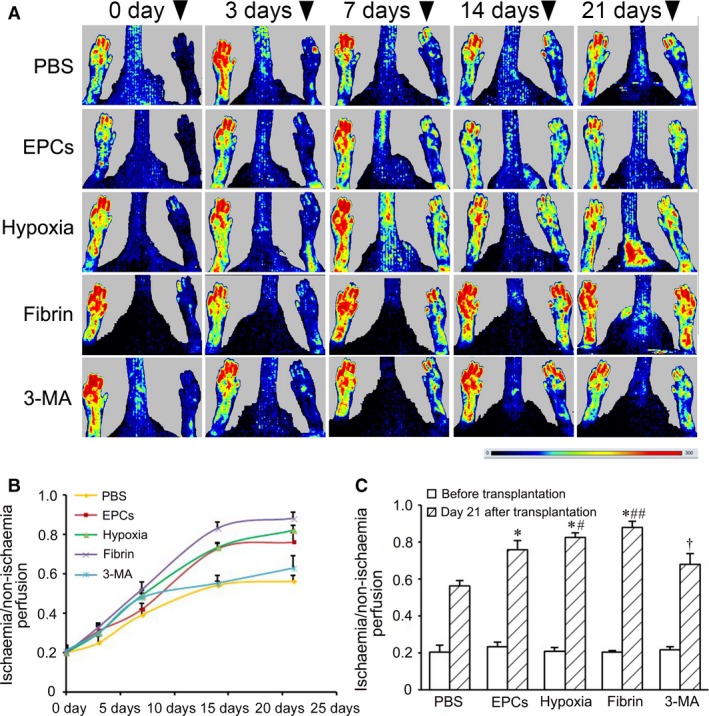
Improvement of blood perfusion in the ischaemic hindlimbs after cell transplantation. (A) Typical images of laser Doppler analysis of blood perfusion. At day 0, 3, 7, 14, 21 after ligation of the femoral artery, blood perfusion in the paws of the hindlimbs was examined with a laser Doppler perfusion image analyser. Arrowheads point to the ischaemic limbs. Bar: dark blue represents low‐to‐no flow, red represents high blood flow. (B and C) Statistic results of blood perfusion at different time‐points (B) and day 21 (C) after cell transplantation (*n* = 4 per group). P* < 0.01 *versus* control group, #*P* < 0.05 and ##*P* < 0.01 *versus *
